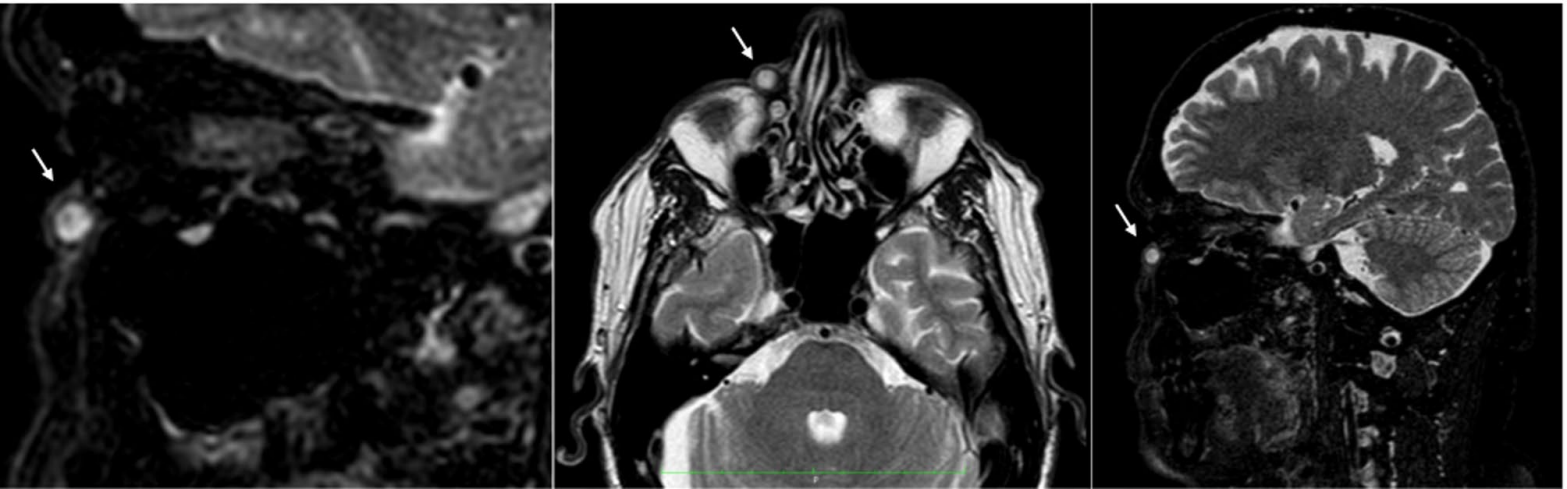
MRI (T2-weighted axial image) round lesion measuring 8 × 8 × 9 millimeters, located in the right inferomedial periorbital area (white arrow), adjacent to the frontal process of the maxillary bone. The lesion was characterized by well-defined and encapsulated margins (thickness of about 2 millimeters), hyperintense signal in T2 with marked enhancement after contrast administration, and a slight faded alteration of the perilesional soft tissue signal, consistent with perilesional edema
